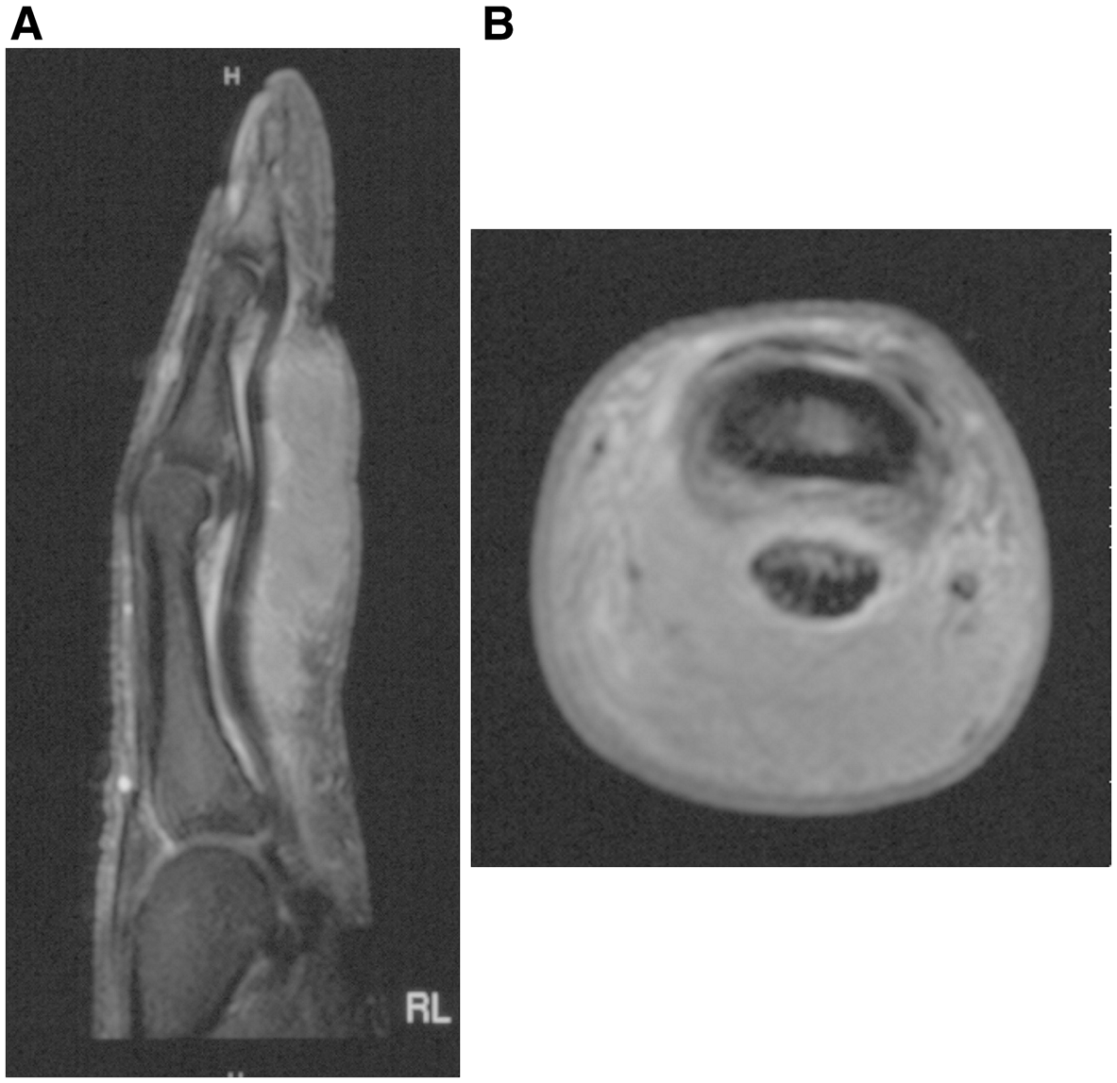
A and B: MRI scans of the right index finger, sagittal (A) and axial (B) slide, T1 with contrast medium. The slides show the size of the swelling based on the long flexor tendon with extension into the dermis. The mass is almost homogenious with a slight enhancement of contrast medium around the tendon. No change in bony structures.
